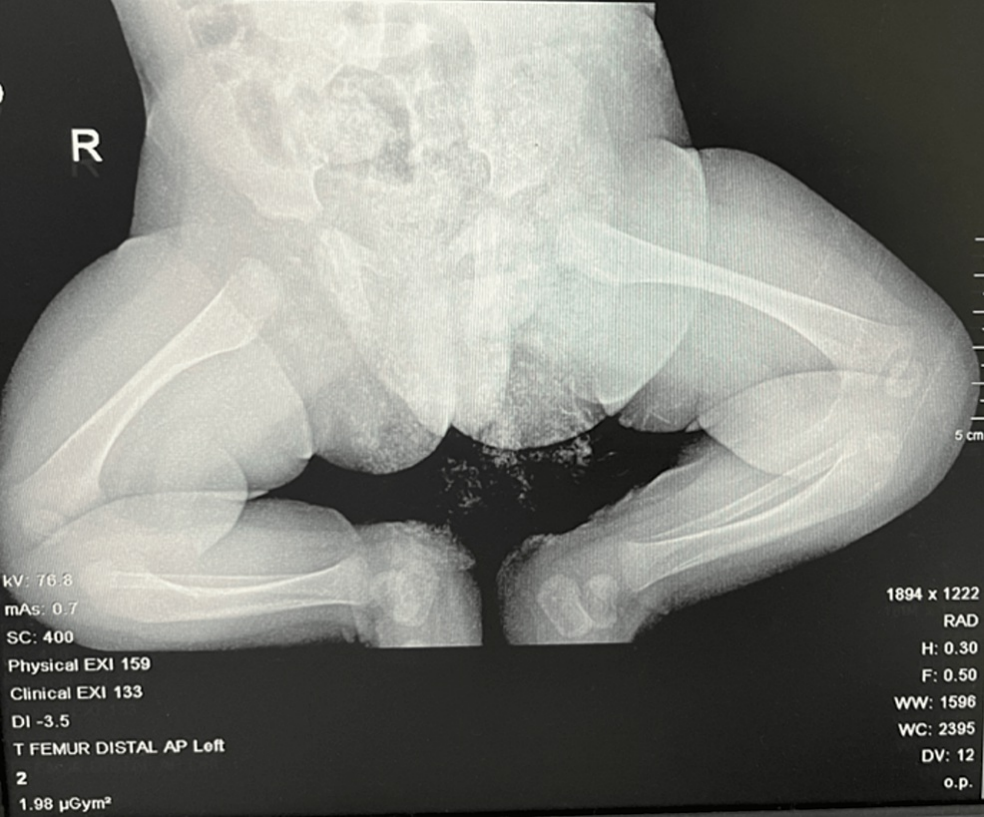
The postoperative hip X-ray (AP view and frog leg lateral view) in an outpatient visit was normal.
